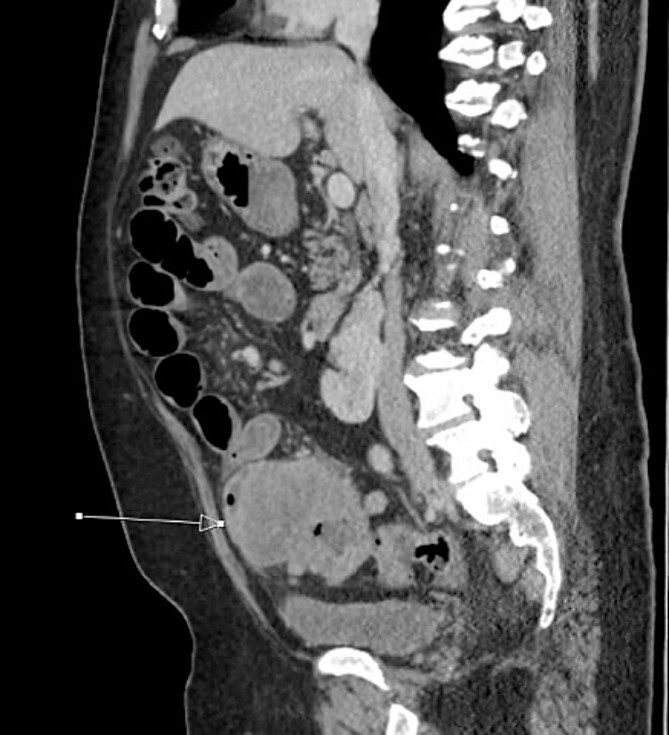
Initial CT imaging, sagittal cut, showing necrotic mass causing small bowel obstruction.
